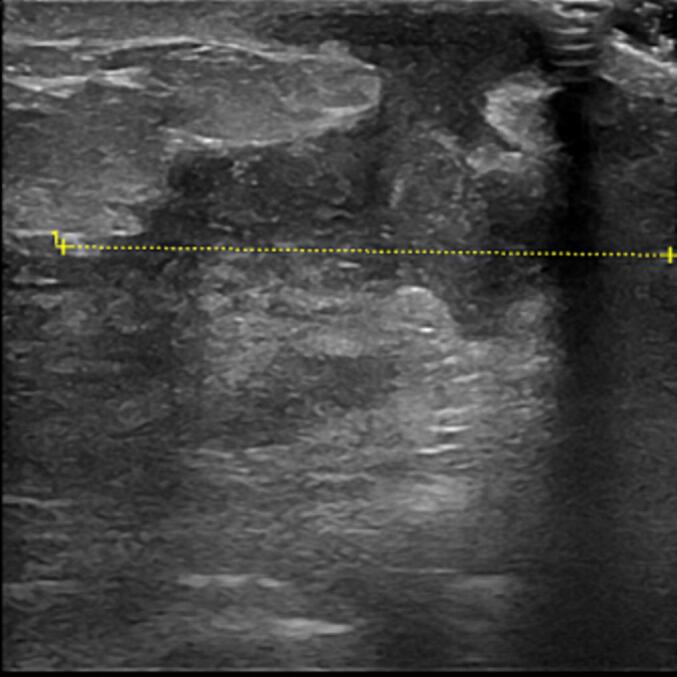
The ultrasound findings showed multiple conflent hypoechoic loculations with ill-defined contours, some of which communicate with the skin, these findings are consistent with mastitis associated with multiple abscessed loculations.
